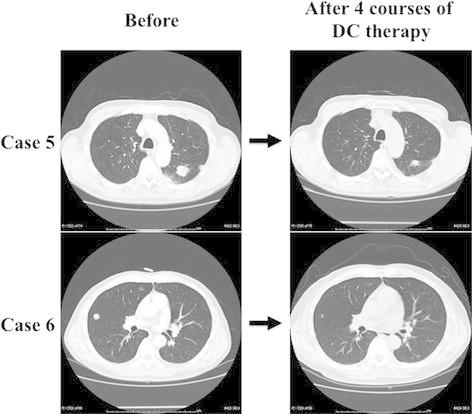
Computed tomography of the lungs in two cases with a partial response. Case 5 showed a 40 % reduction in the tumor volume from baseline. Case 6 showed a near-complete response
